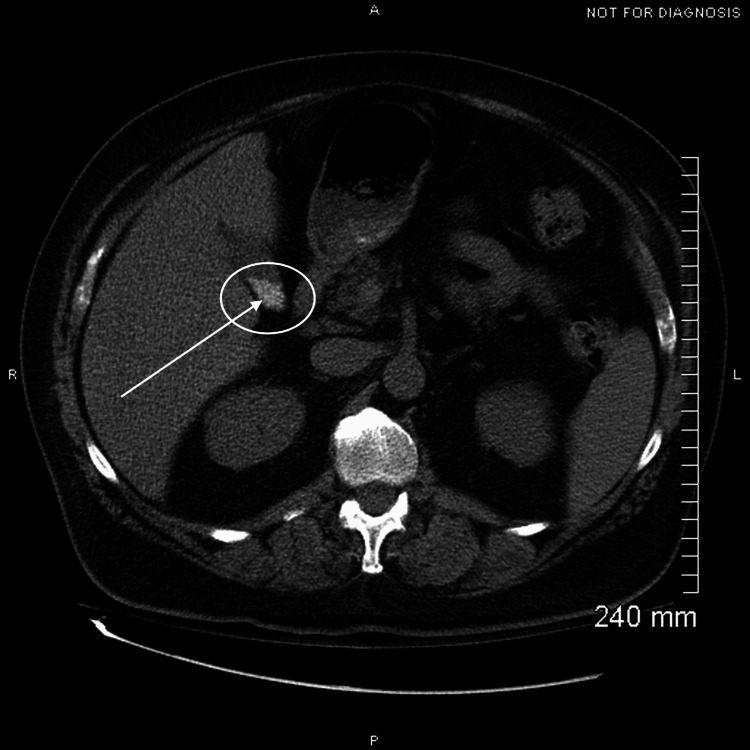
Axial CT of the abdomen/pelvis showing a hyperdense focus within the gallbladder consistent with gallstones. No wall thickening, pericholecystic fluid, or ductal dilation is present.
